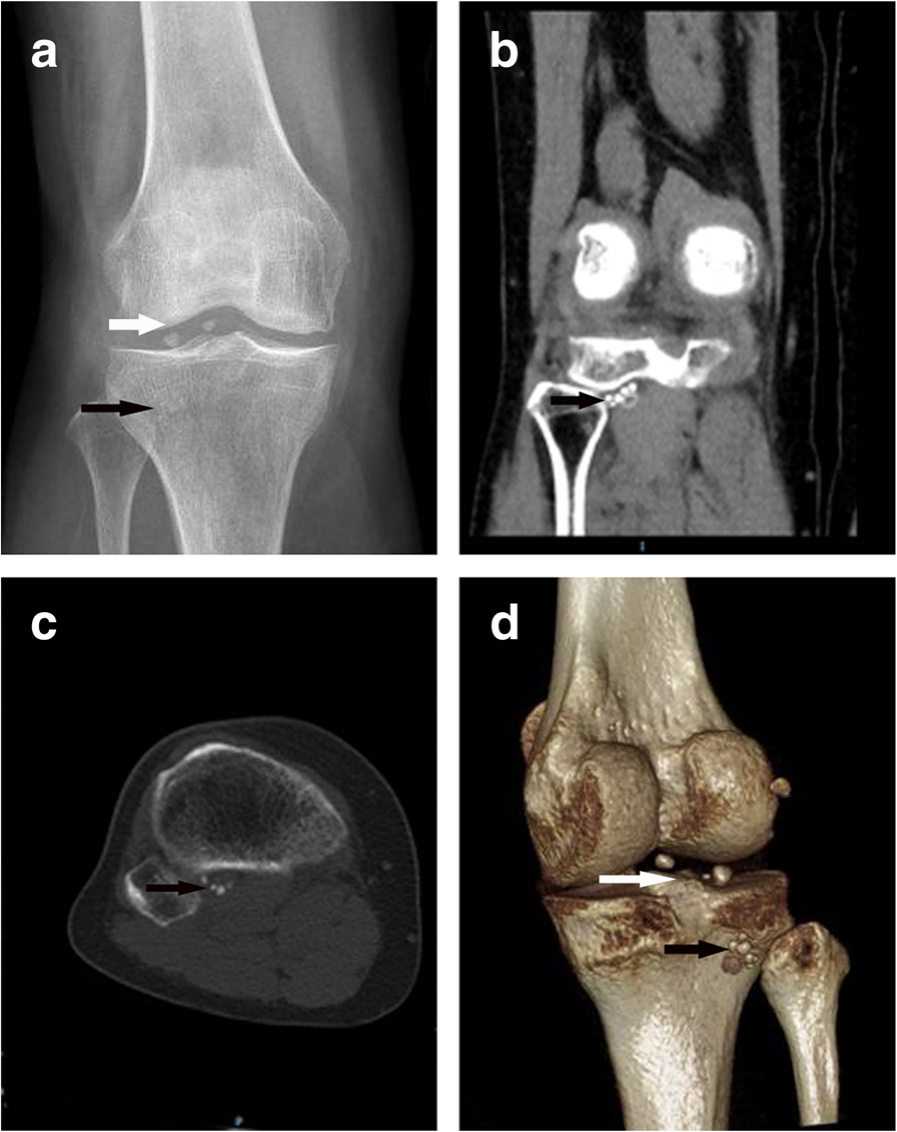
Images of loose bodies in the popliteus hiatus of the knee. Several ones (black arrows) were on the medial side of the fibular head, the other two (white arrows) were at the joint space. a A preoperative anteroposterior radiograph. b A coronal view of MRI. c, d Horizontal and three-dimensional rebuilding views of CT, respectively
